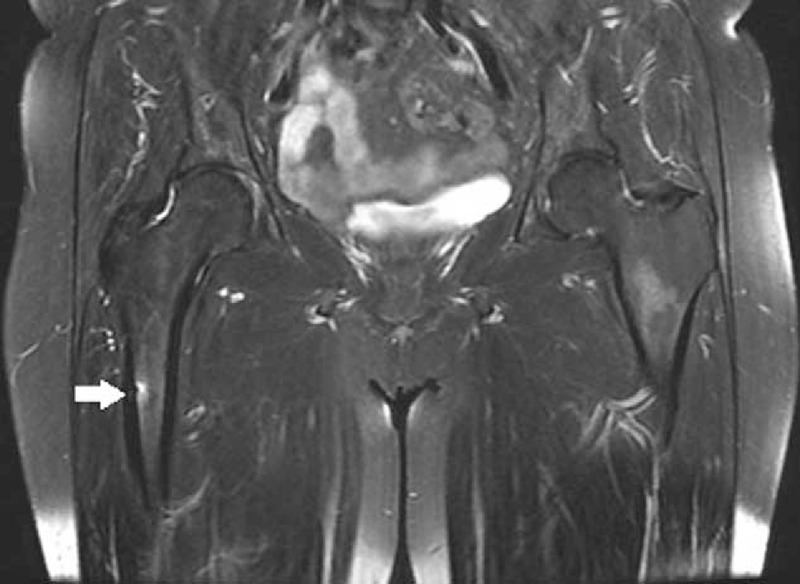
Hip MRI reveals a right femur subtrochanteric fracture (arrows). MRI = magnetic resonance imaging.
